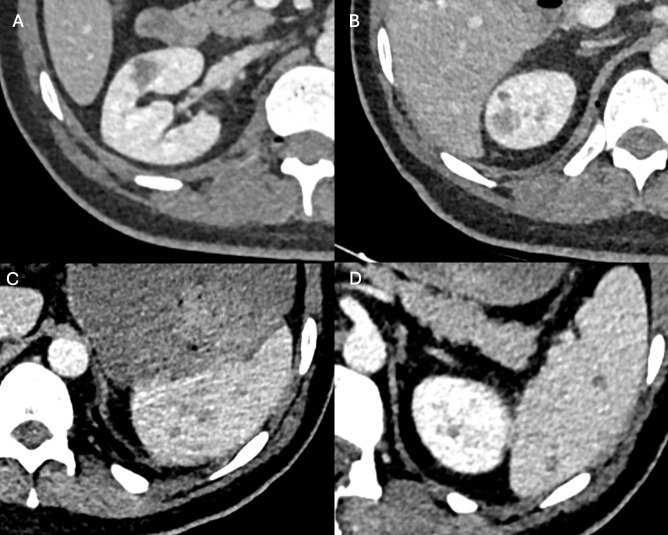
Axial contrast-enhanced CT of the abdomen demonstrates multiple small, ill-defined hypodense lesions within the right kidney (A, B) and spleen (C, D), suspicious for septic emboli.
